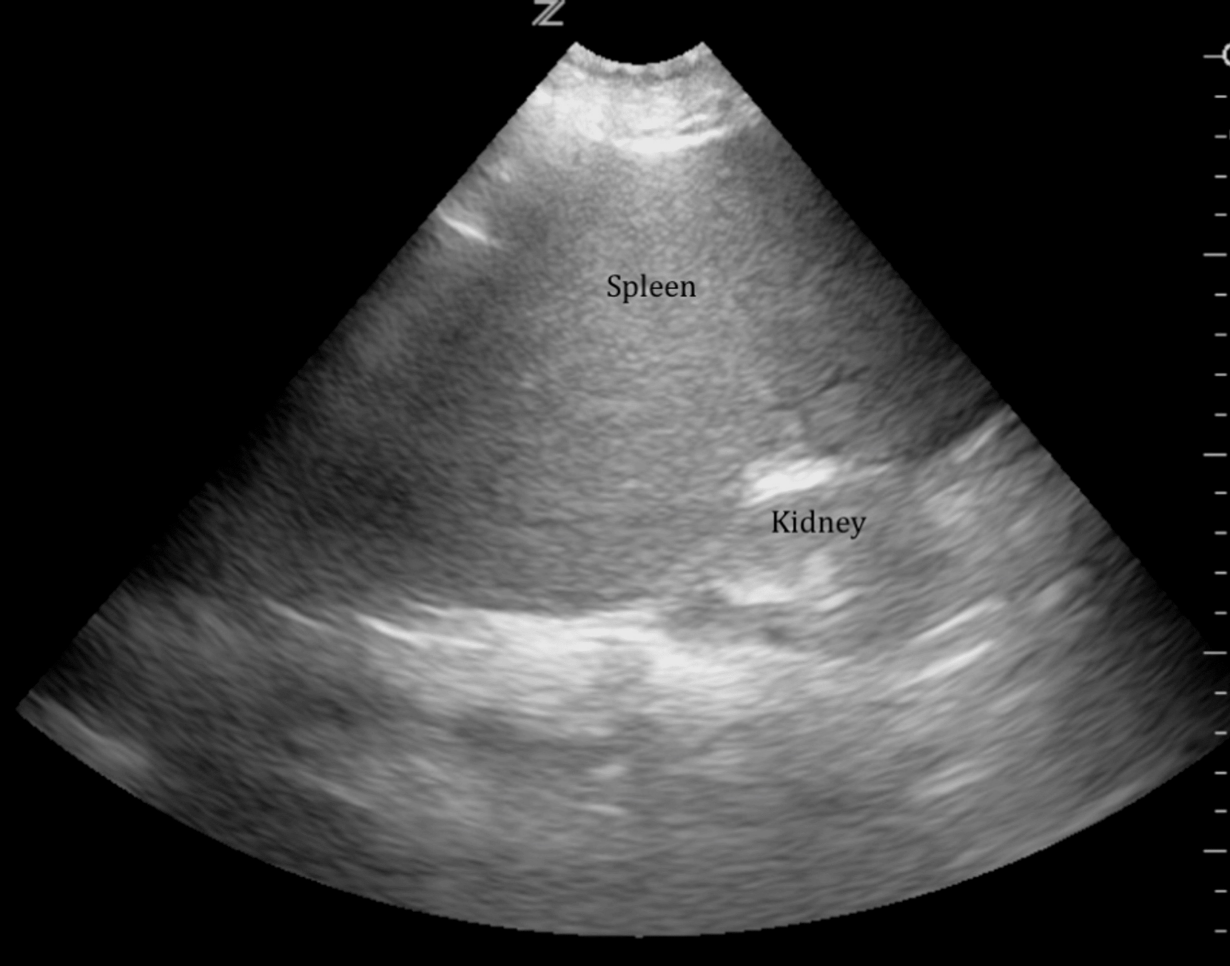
Abdominal ultrasonography Ultrasonography image showing a normally sized spleen with no evidence of splenomegaly or focal lesions.
